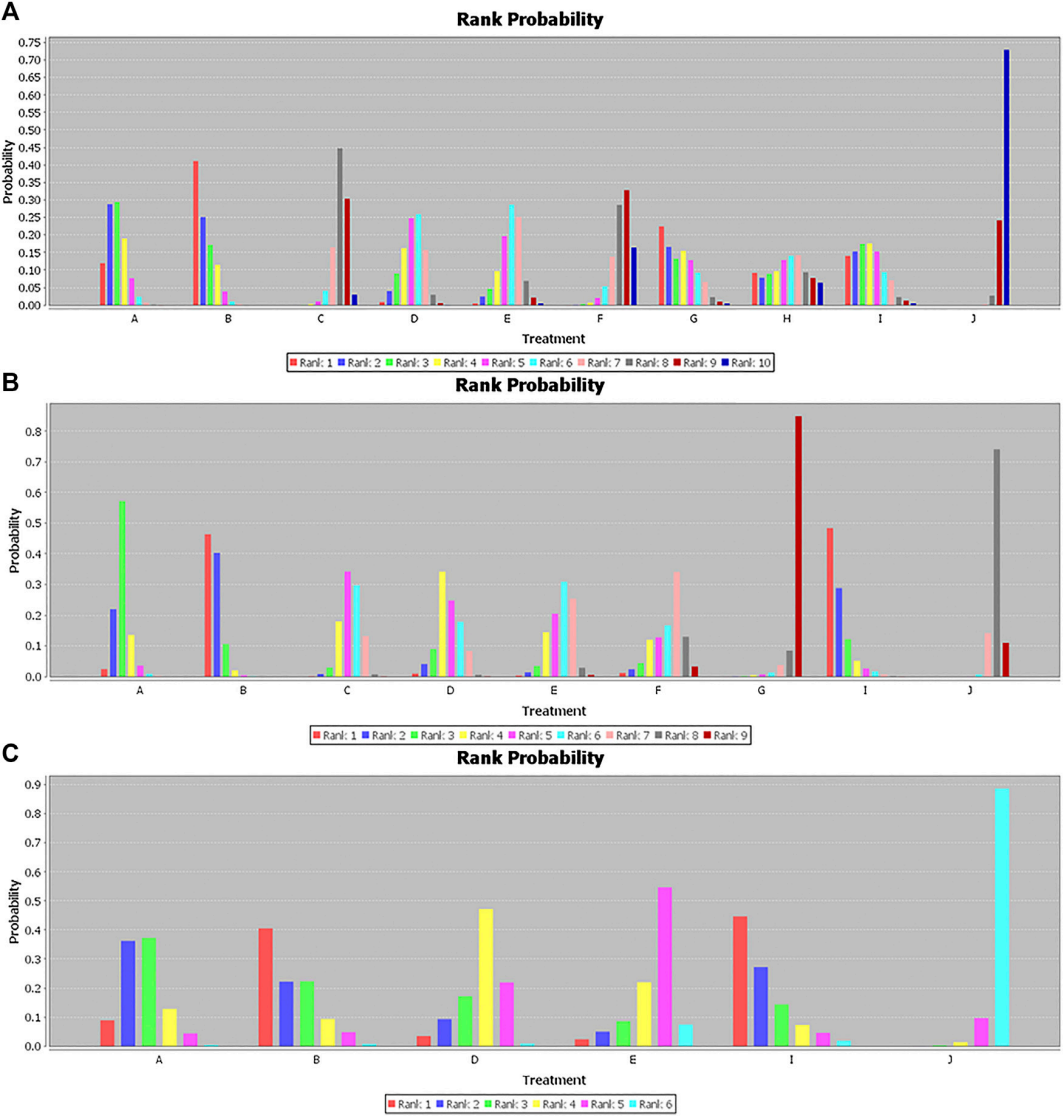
Rank probability for (A) induction of remission. (B) Maintenance of remission. (C) Steroid-free remission. A, Infliximab; B, Adalimumab; C, Certolizumab pegol; D, Vedolizumab; E, Ustekinumab; F, Tofacitinib; G, Filgotinib; H, Upadacitinib; I, CT-P13; J, Placebo.
